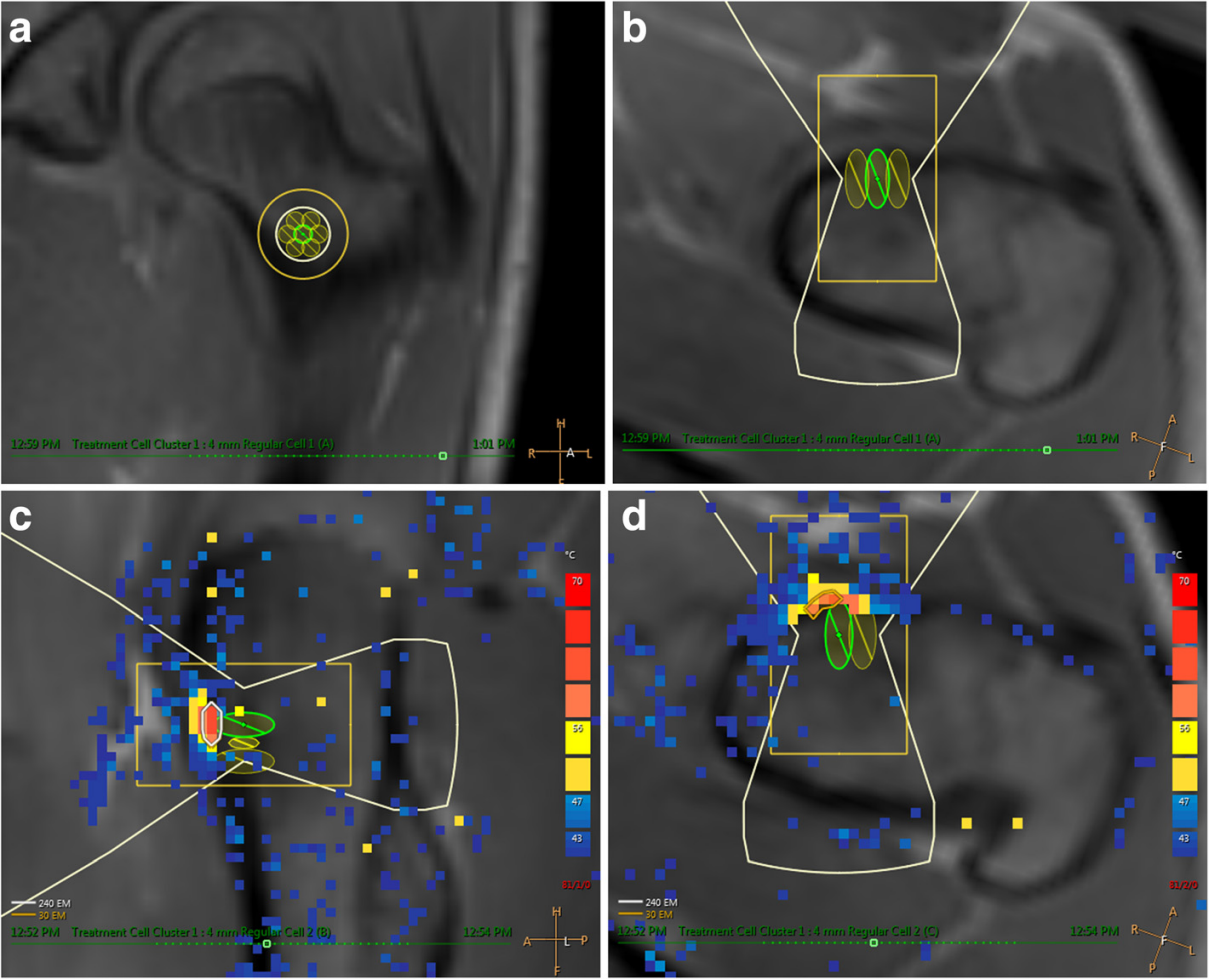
Thermography during osteoid osteoma treatment. Planned treatment cells for the osteoid osteoma from Fig. 1 shown in a coronal and b axial MRI views on a Philips Sonalleve platform. (User interface not shown). Seven 4-mm treatment cells were arranged in a circular cluster in the coronal plane to cover the entire 1-cm lesion. Due to electronic beam steering on the Sonalleve machine, the focal zone was positioned at the bone surface in the osteoid osteoma. c Sagittal and d axial images demonstrate thermal maps from a 50-W (1000 J) treatment sonication. This exposure produced a maximum temperature above 60 °C at the bone surface. A small region (approximately 1 × 4 mm) adjacent to the osteoid osteoma reached sufficient temperatures to achieve a thermal dose of 240EM@43 °C, causing necrosis.
